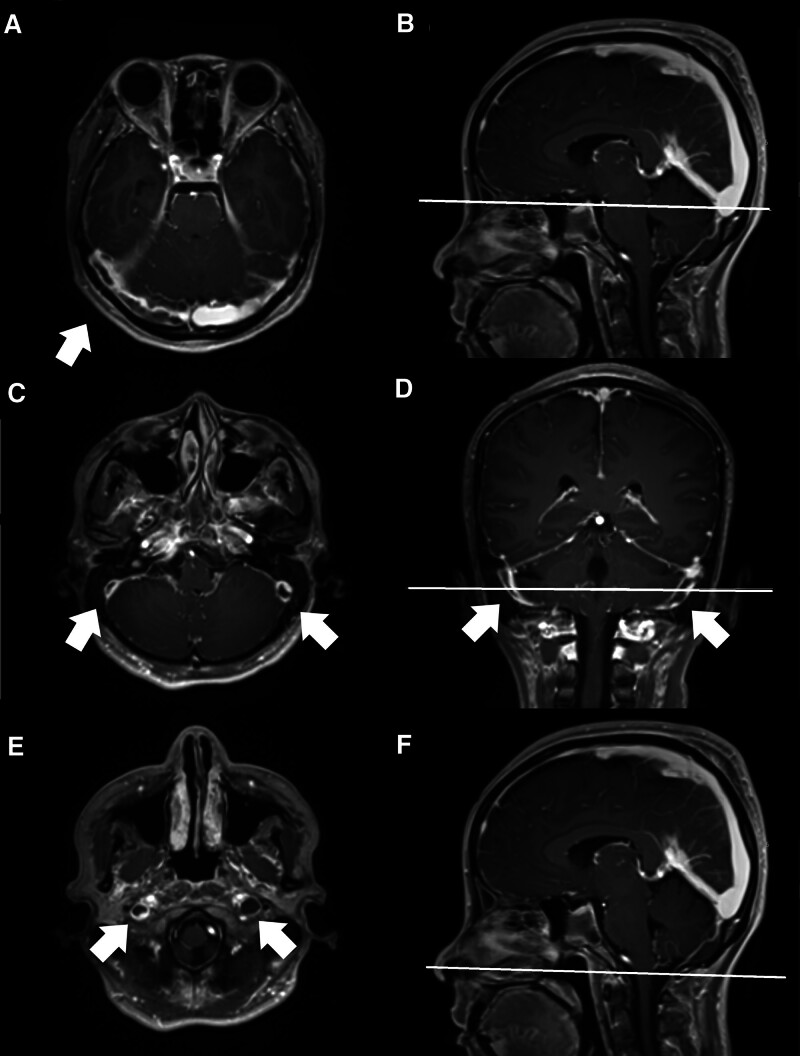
MRI scan of brain T1 image with contrast medium of a 33-yr-old woman reveals cerebral venous sinus thrombosis in the right transverse sinus, bilateral sigmoid sinuses, and bilateral internal jugular veins. (A) A transverse view of the brain MRI demonstrates a thrombus in the right transverse sinus. (B) A sagittal view of the brain MRI shows a hyperintense signal in the superior sagittal and straight sinuses. The white line indicates the image level of (A). (C) A transverse view of the brain MRI demonstrates the absence of flow in the bilateral sigmoid sinuses. (D) A coronal view of the brain MRI shows a thrombus in the bilateral sigmoid sinuses. The white line indicates the image level of (C). (E) A transverse view of the brain MRI demonstrates a thrombus in the bilateral internal jugular vein. (F) The transverse line in the sagittal view of the brain MRI scan indicates the image level of (E). MRI = magnetic resonance image.
